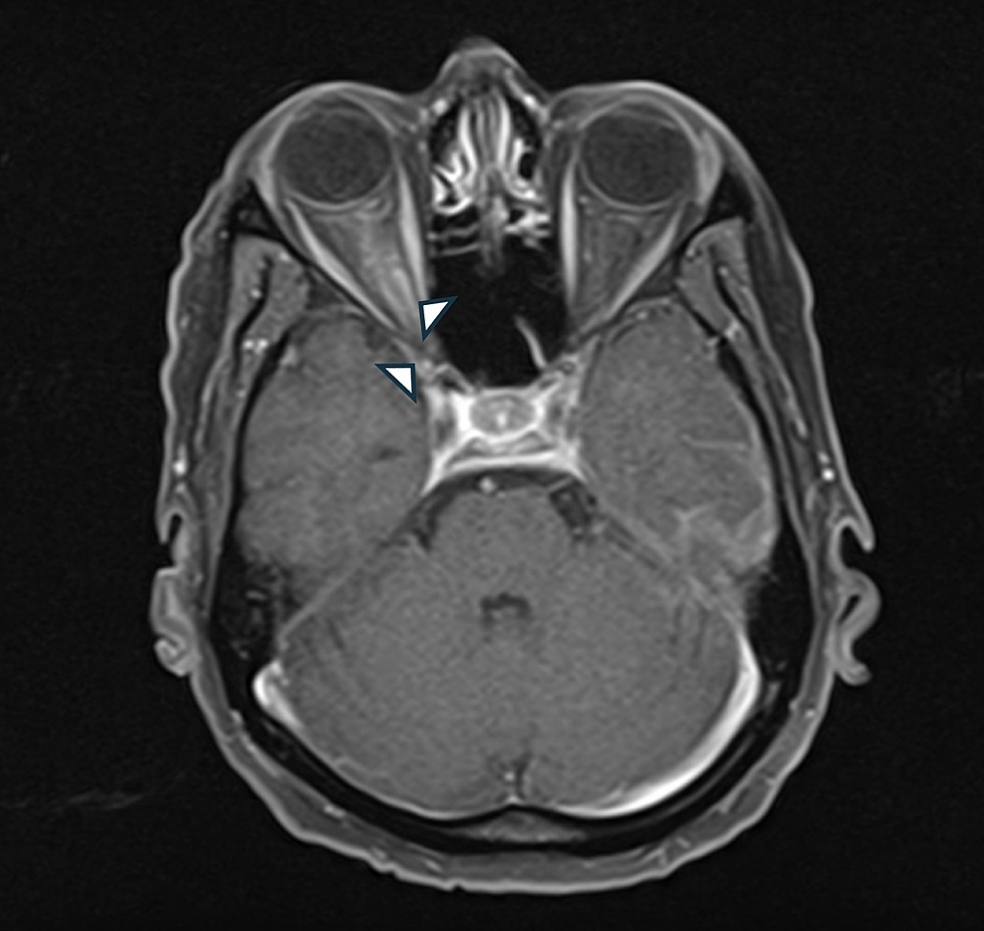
Arrowheads: axial T1-weighted gadolinium-enhanced MRI demonstrates contrast enhancement of the pre-chiasmatic and canalicular and orbital parts of the right optic nerve
